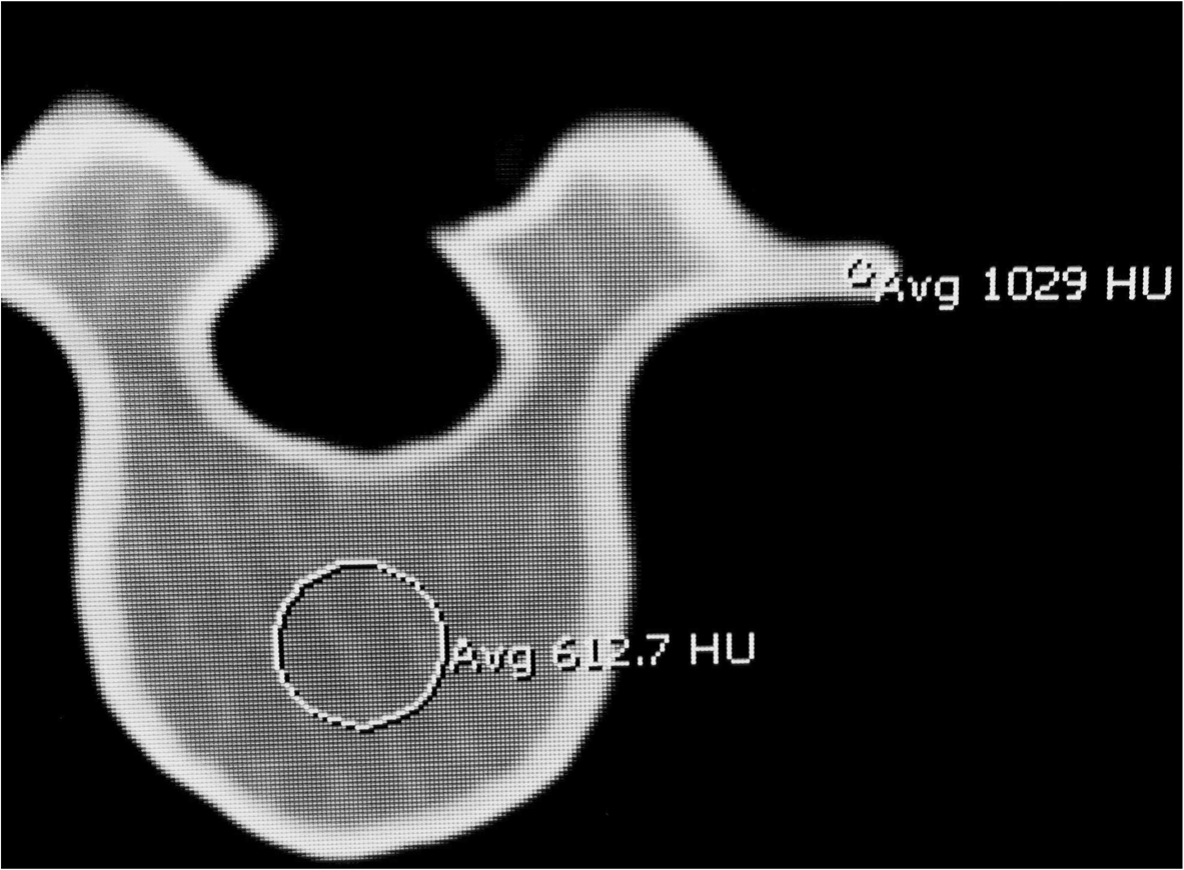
Under CT, the lumbar spine model demonstrates Hounsfield Units that mimic cortical and medullary bone
